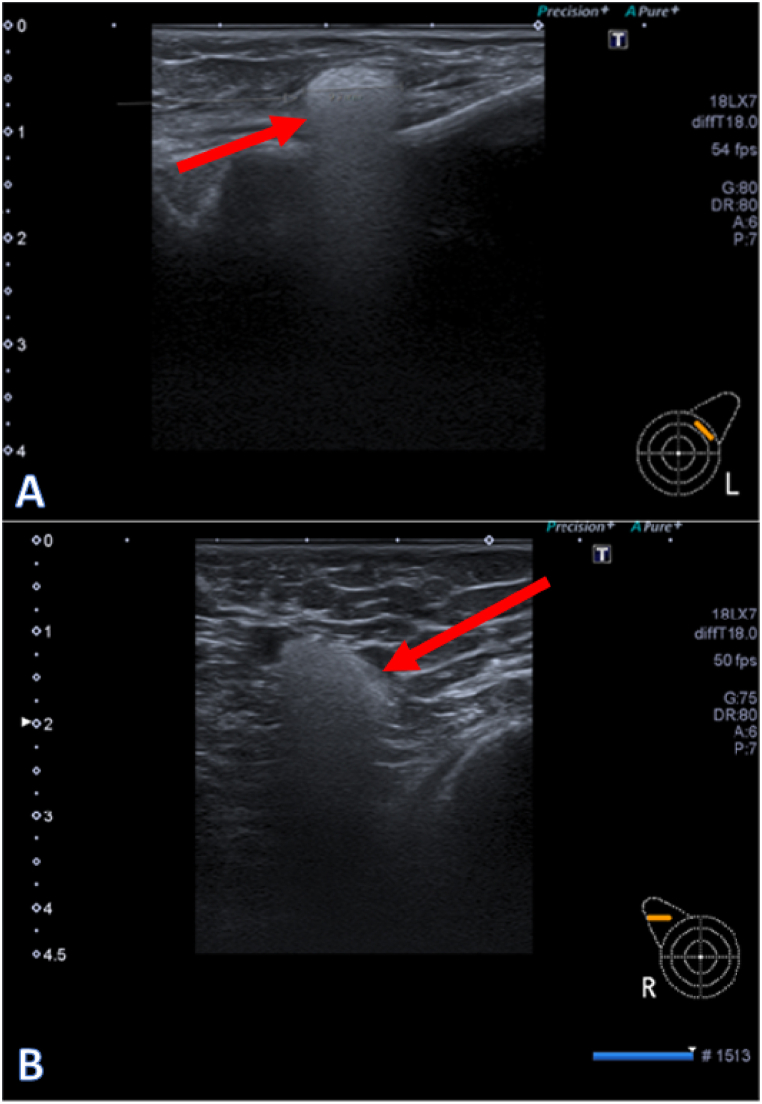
Ultrasound of silicone depositions. A.Ultrasound of the left breast showing a silicone deposition. B. Ultrasound showing a lymph node with evident snowstorm sign in the right axilla, indicating silicone depositions.
